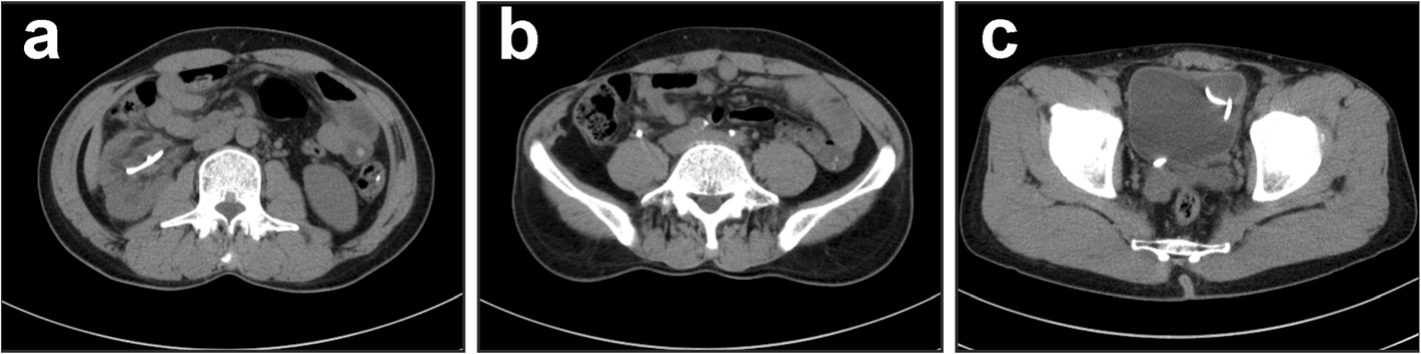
The computed tomography scan is performed 3 months after surgery. a, b, and c Axial images showing that no residual fragments are found
